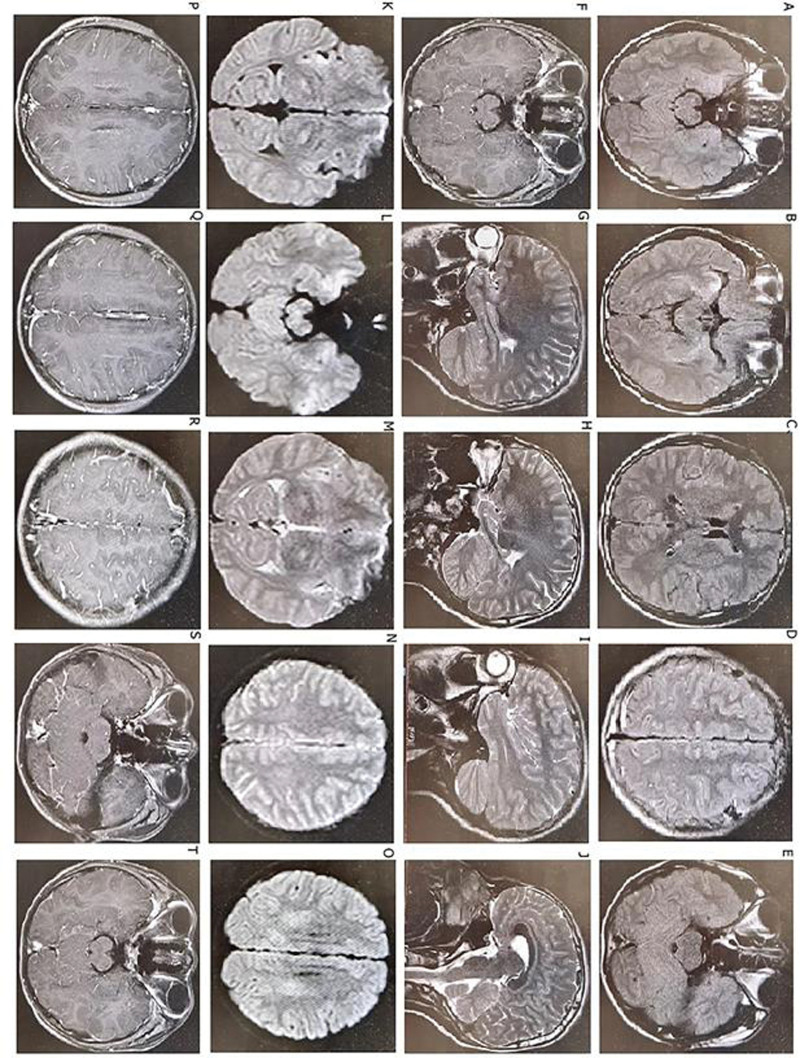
The results of cranial MRI (plain scan + enhanced + MRA + MRV). The findings revealed that the lesions of the right hippocampus, amygdala, and insular lobe and local sulcus enhancement of the right insular, temporal, and frontal lobes were enhanced using FLAIR. (A–E) T2 FLAIR image. (K–O) Diffusion-weighted image. (F–J and P–T) T1-weighted image. FLAIR = fluid-attenuated inversion recovery, MRA = magnetic resonance angiography, MRV = magnetic resonance venography.
